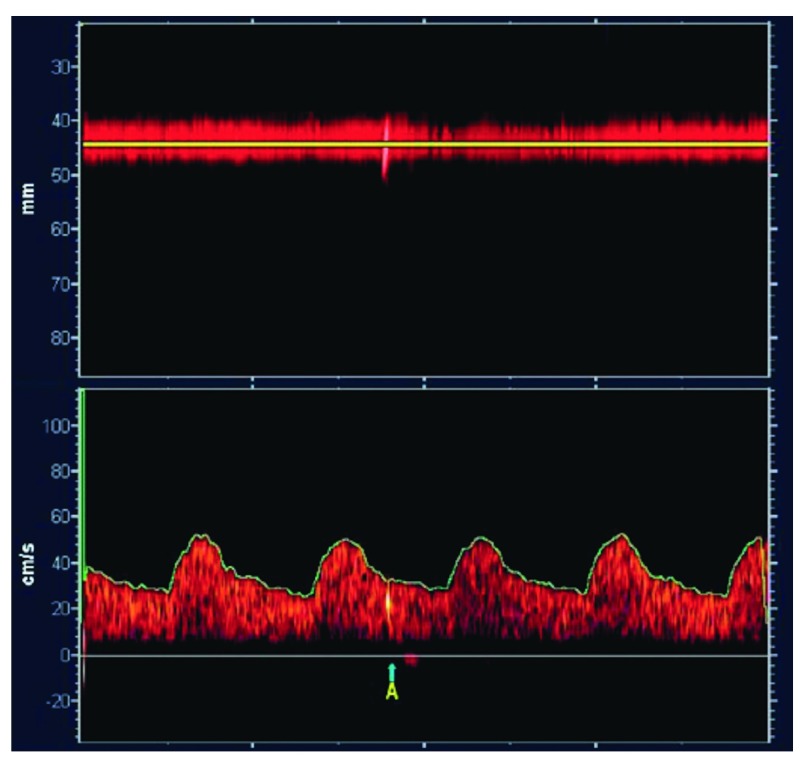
Transcranial Doppler embolus detection. Microembolus in a patient with asymptomatic carotid stenosis. The upper channel is an M-mode image of an embolus in the middle cerebral artery; the lower panel shows the high-intensity transit signal in the Doppler channel. Besides the visual appearance of the microembolus, a characteristic clicking sound is heard. Reproduced by permission of the Society for Vascular Ultrasound from: Spence JD. Transcranial Doppler: uses in stroke prevention. The Journal for Vascular Ultrasound 2015, 39(4): 183–187.
